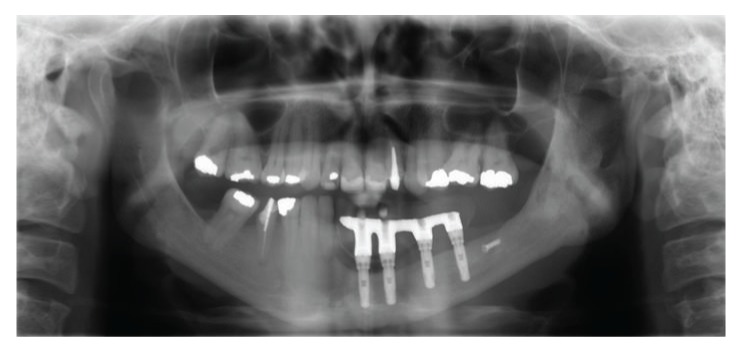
Panoramic radiographic 6 years after tumor resection.
